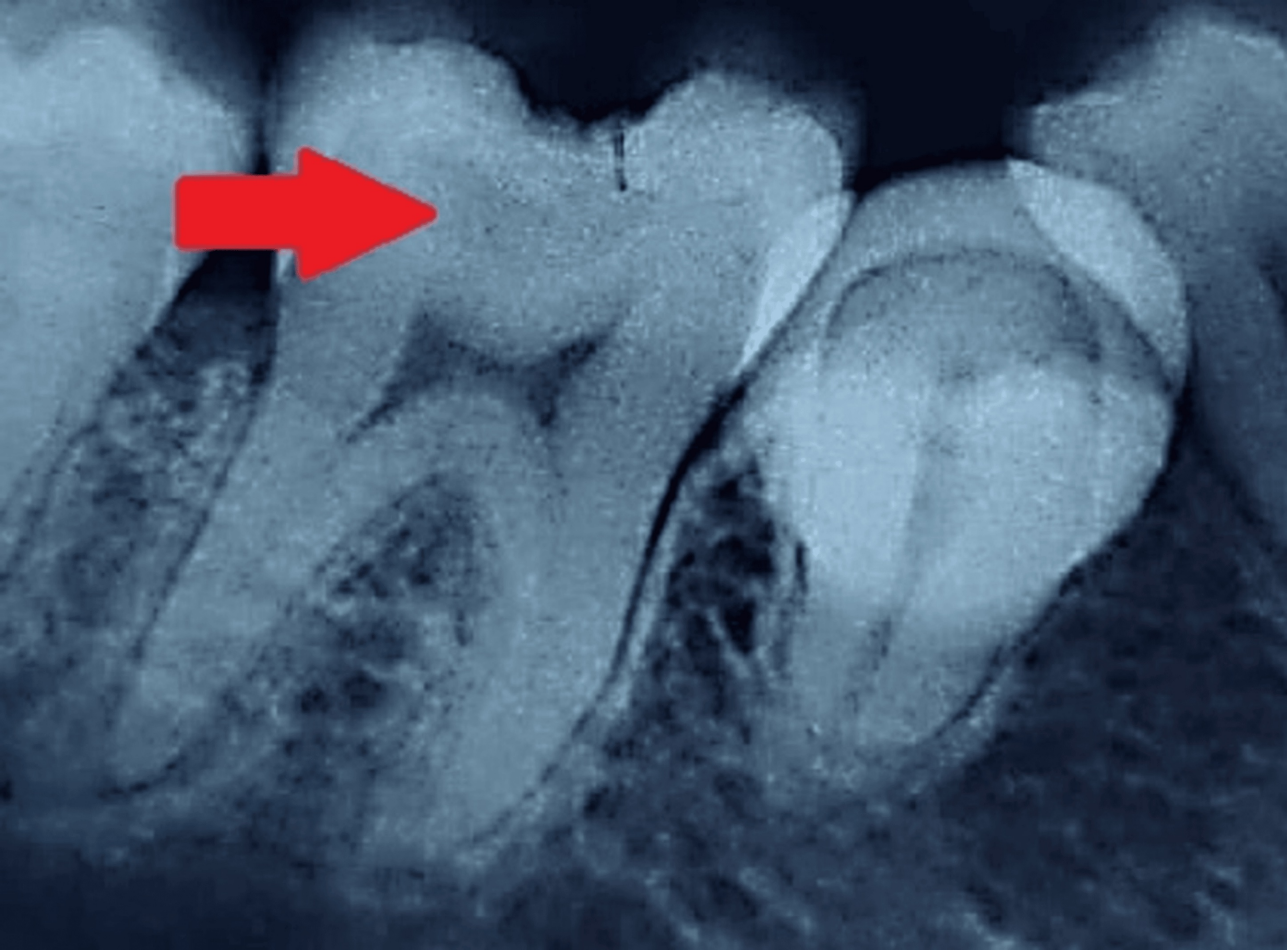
Preoperative intra-oral periapical radiograph showing deep carious lesion in the lower right mandibular first molar The arrow shows radiolucency involving enamel, dentin, and approaching pulp.
